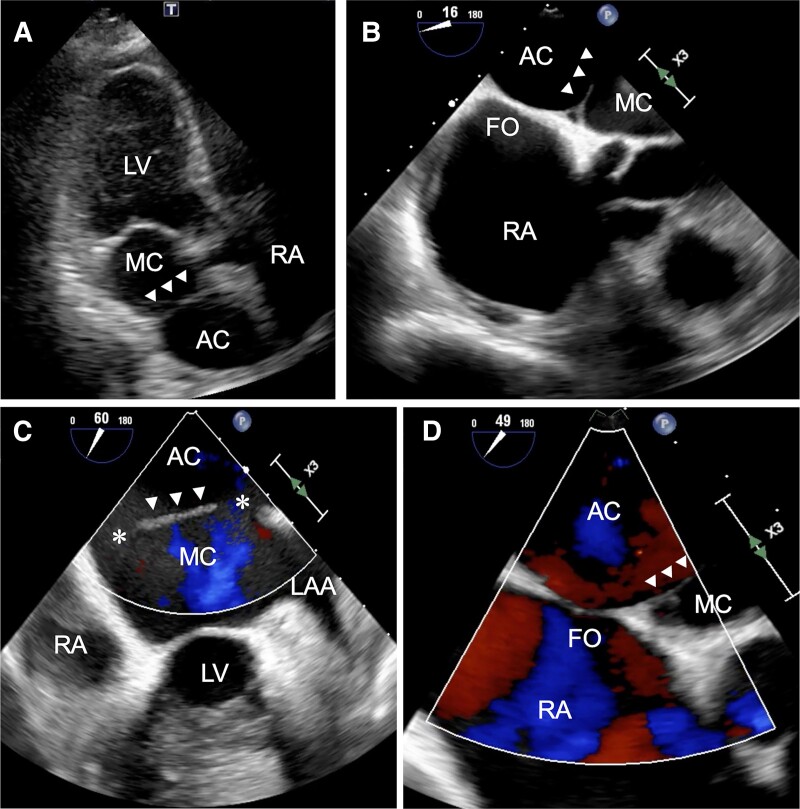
Echocardiography findings. (*A*) Transthoracic echocardiography. (*B* and *C*) Transoesophageal echocardiography: the left atrium is divided into two chambers by a membrane. (*D*) Transoesophageal echocardiography: the membrane has two orifices (*). LAA, left atrial appendage; RA, right atrium; LV, left ventricle; FO, fossa ovalis; AC, accessory chamber; MC, main chamber; TTE, transthoracic echocardiography; TEE, transoesophageal echocardiography. Arrow heads, membrane.
